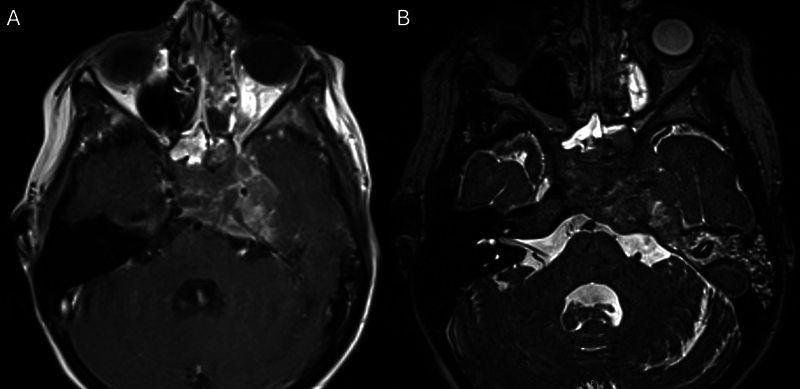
Repeat MRI at 2.5 weeks (
